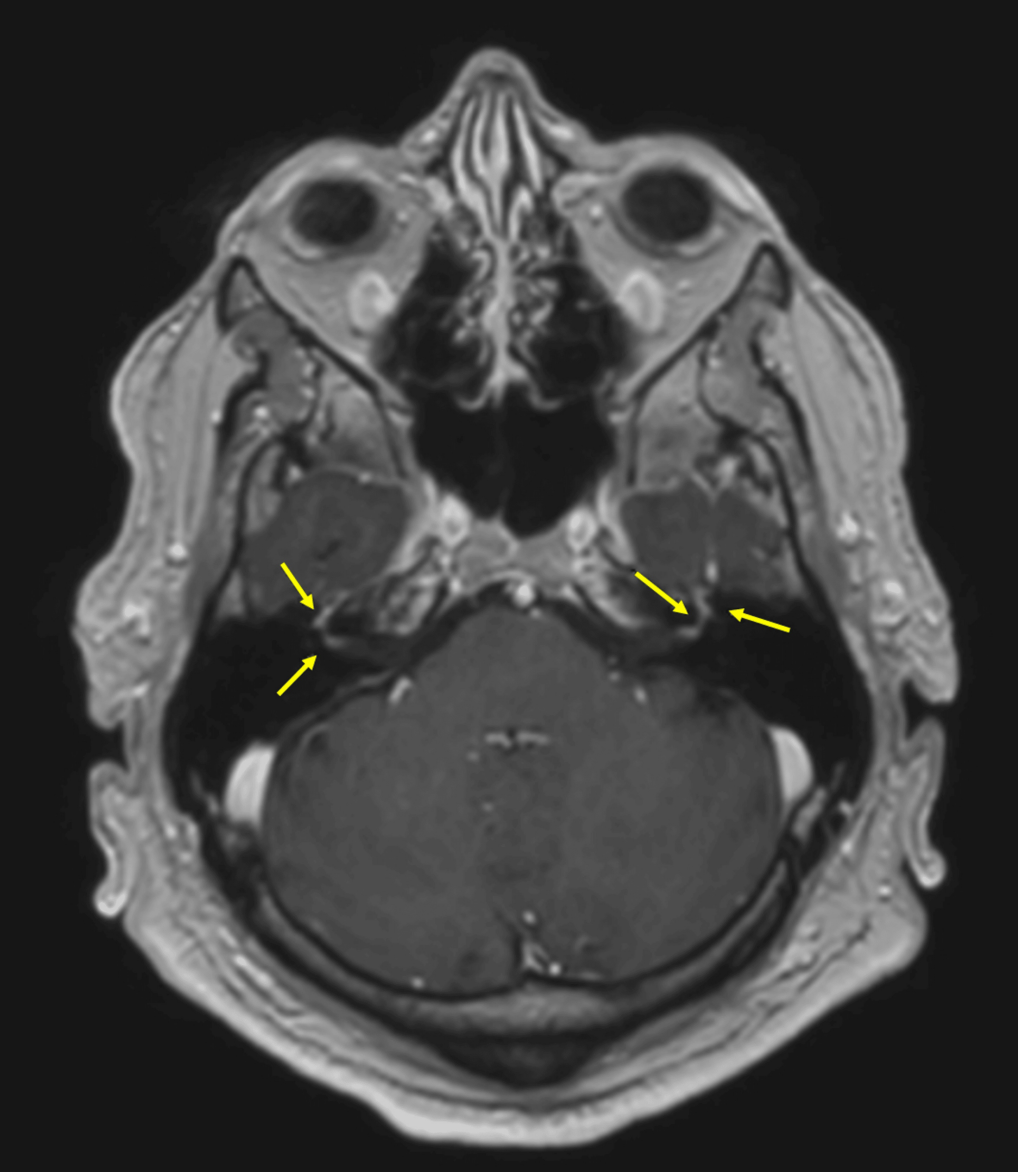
Axial section of MRI of the head with contrast showing pathologic enhancement at the fundus of both IAMs (yellow arrows). IAMs: internal acoustic meatuses.
